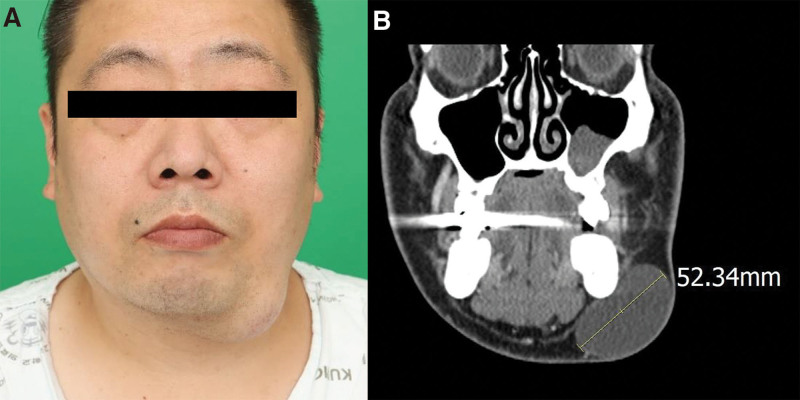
(A) Case 10: A rare case of UGEC located on the left chin. (B) Coronal computed tomography showing a 5.2 × 4.3 × 2.8 cm^3^ UGEC. UGEC = unilocular giant epidermal cyst.
